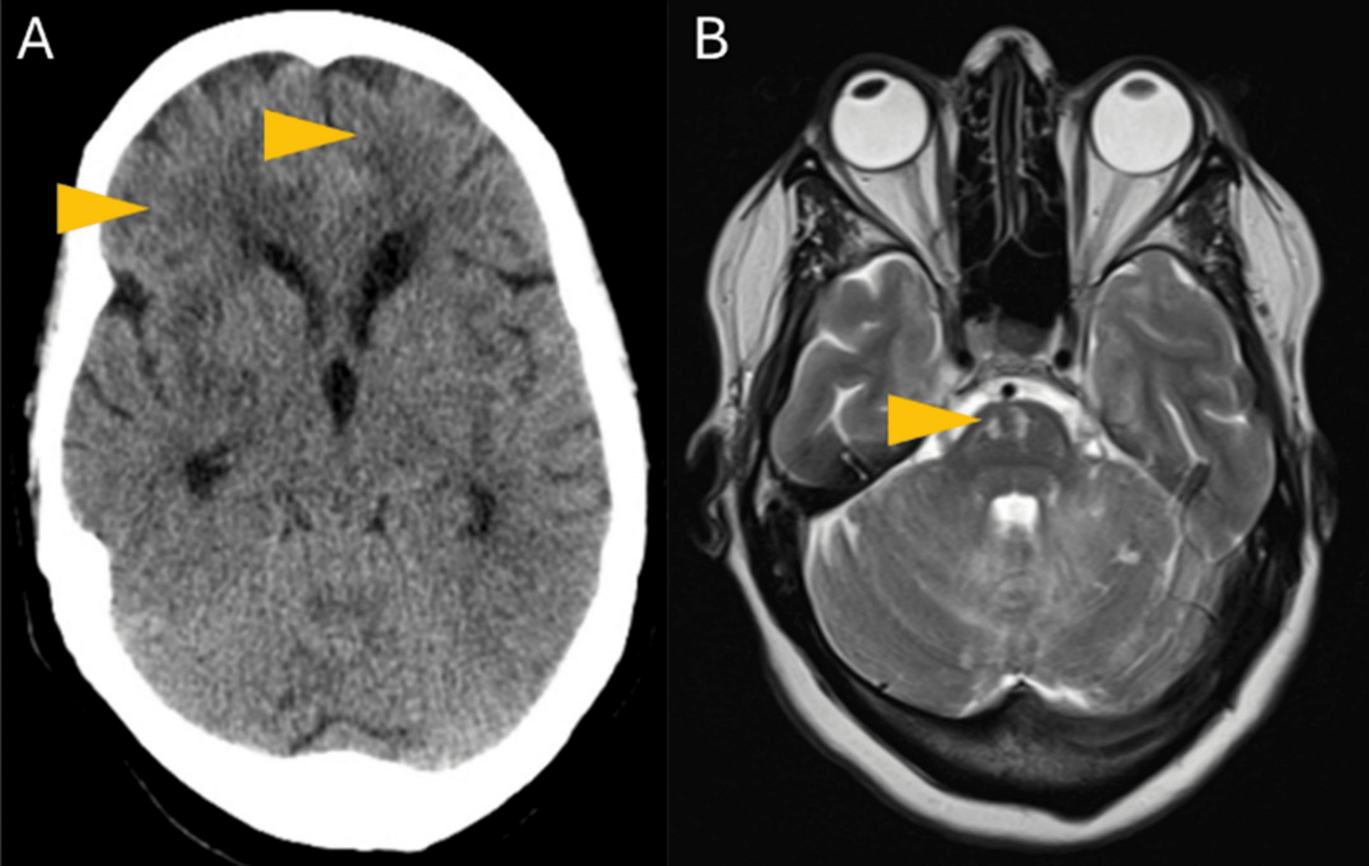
Brain CT and MRI images. A computed tomography (CT) scan in the axial view revealed anterior bulging hypodensities, more evident in the right hemisphere (A). T2-weighted magnetic resonance imaging (MRI) in the axial view showing an acute ischemic injury in the right basilar artery territory (B).
